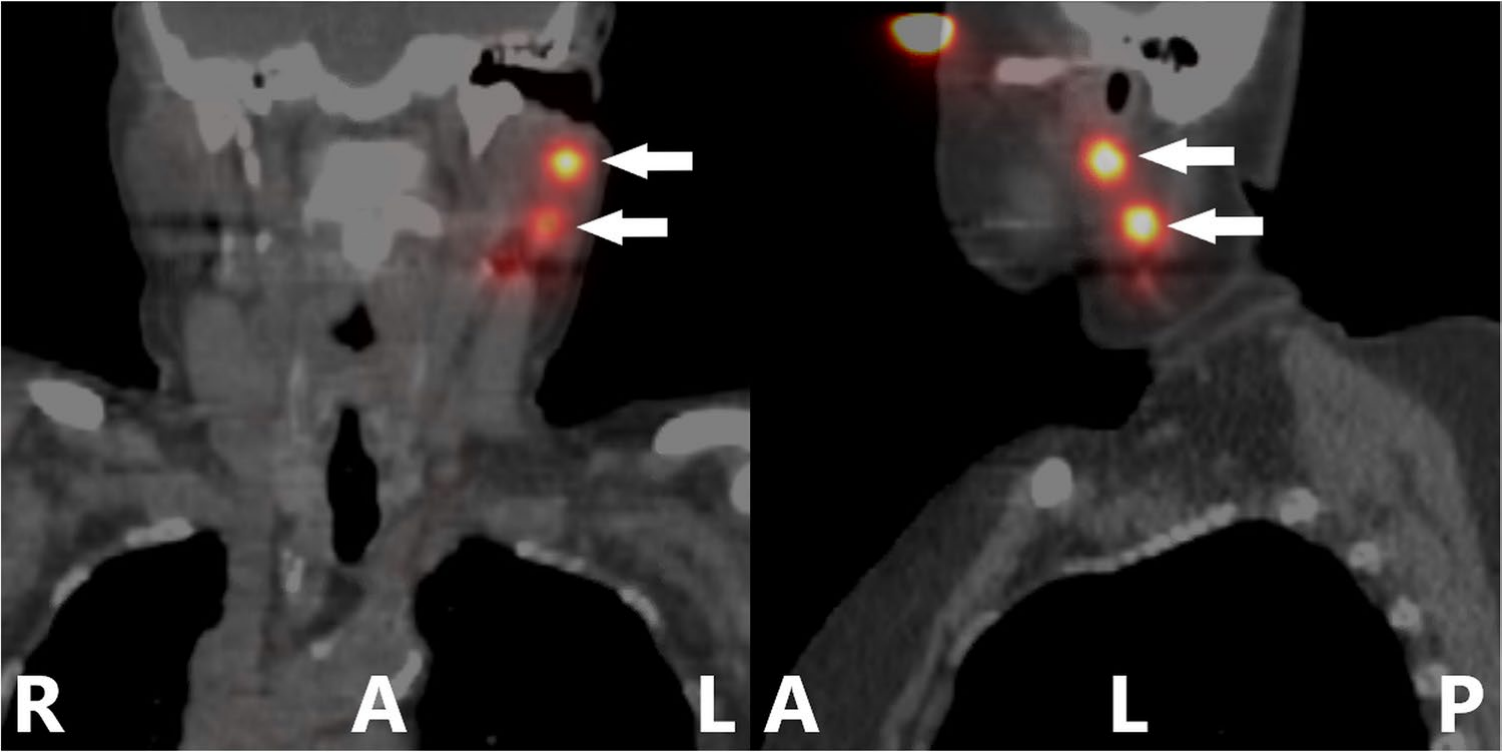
Single-photon emission computed tomography/computed tomography. Single-photon emission computed tomography/computed tomography in coronal projection (left) and sagittal projection (right) of a patient with a superior periocular primary tumour 2 h after injection of [^99m^Tc]Tc-tilmanocept; localisation of two SLNs (arrows); R, right patient side; L, left patient side; A, anterior patient side; P, posterior patient side [artwork modified with Microsoft Paint, Microsoft, Redmond, USA]
